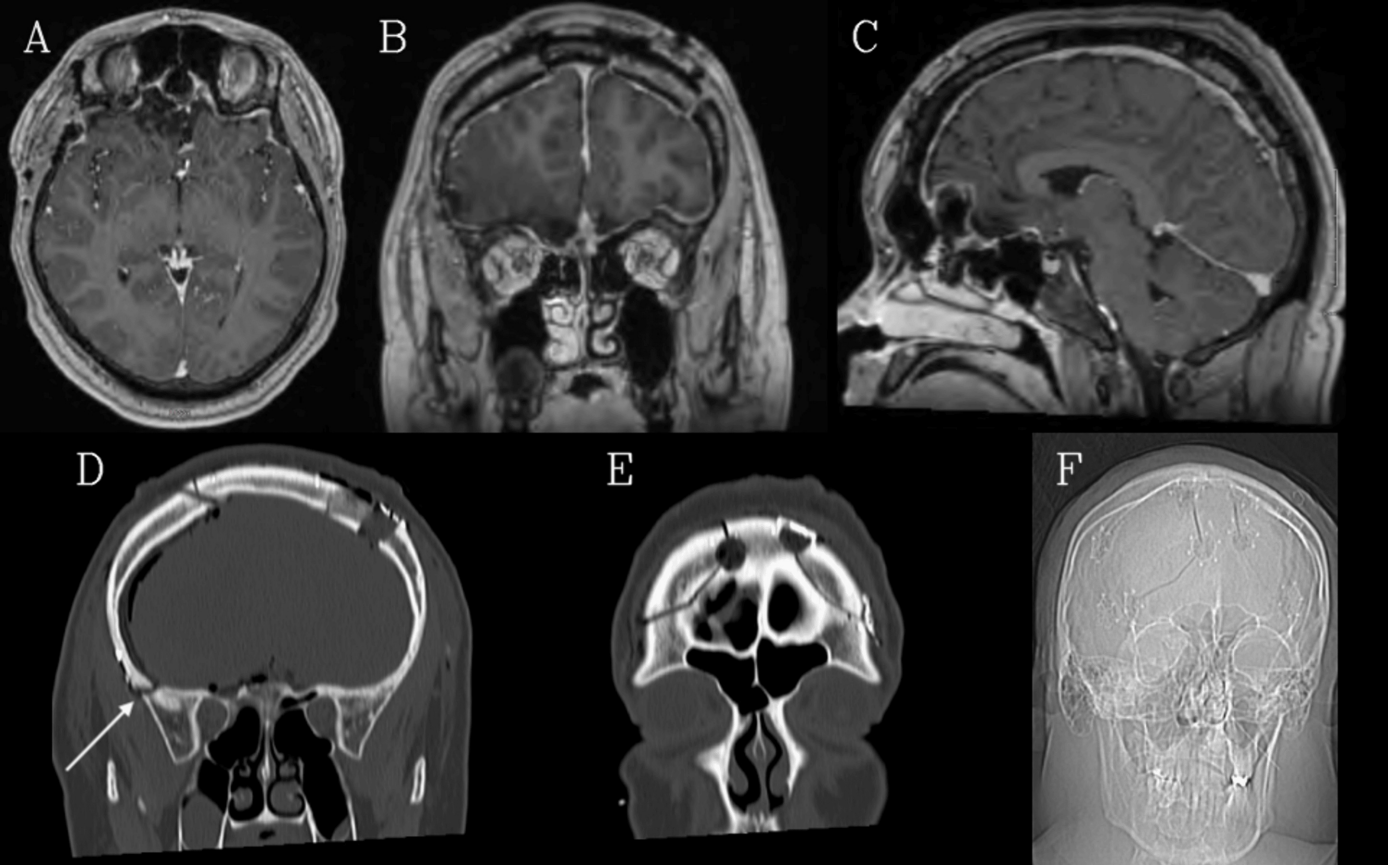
Postoperative imaging A, B, C: Axial, coronal, and sagittal T1 contrasted images, respectively, demonstrate near-total resection of the mass. D, E, F: Coronal CT (bone window and accompanying scout image) showing the outlines of bilateral frontal craniotomies, with the right-sided one extending more inferiorly towards the skull base, given that the mass was predominantly on the right (arrow).
